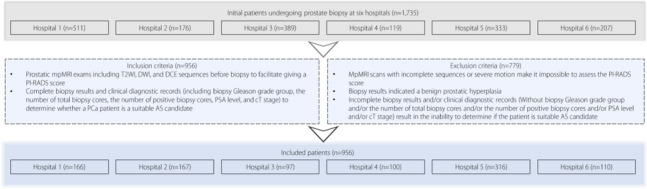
Overview of patients based on the inclusion and exclusion criteria and allocation of patients in the training and external validation cohorts. cT stage: clinical tumor stage; DCE: Dynamic contrast-enhanced; DWI: Diffusion-weighted imaging; mpMRI: Multiparametric magnetic resonance imaging; PSA: Prostate-specific antigen; T2WI: T2-weighted imaging
